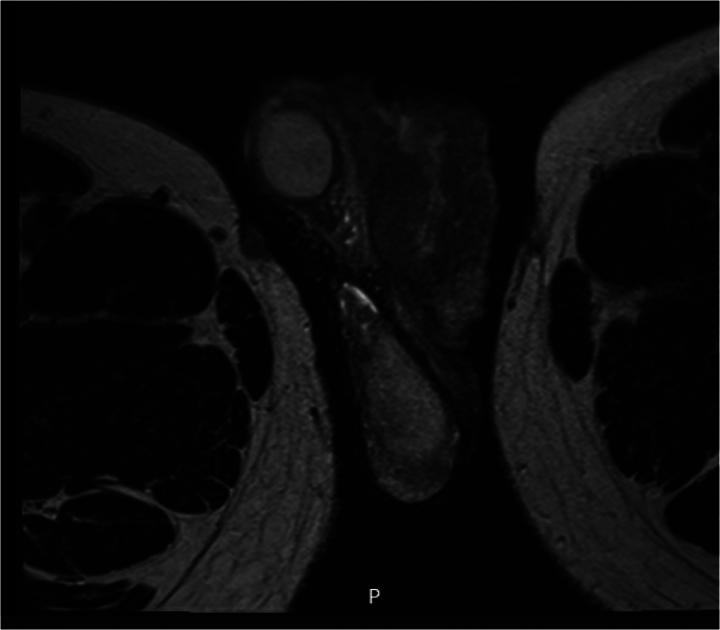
Axial T2-weighted magnetic resonance image
